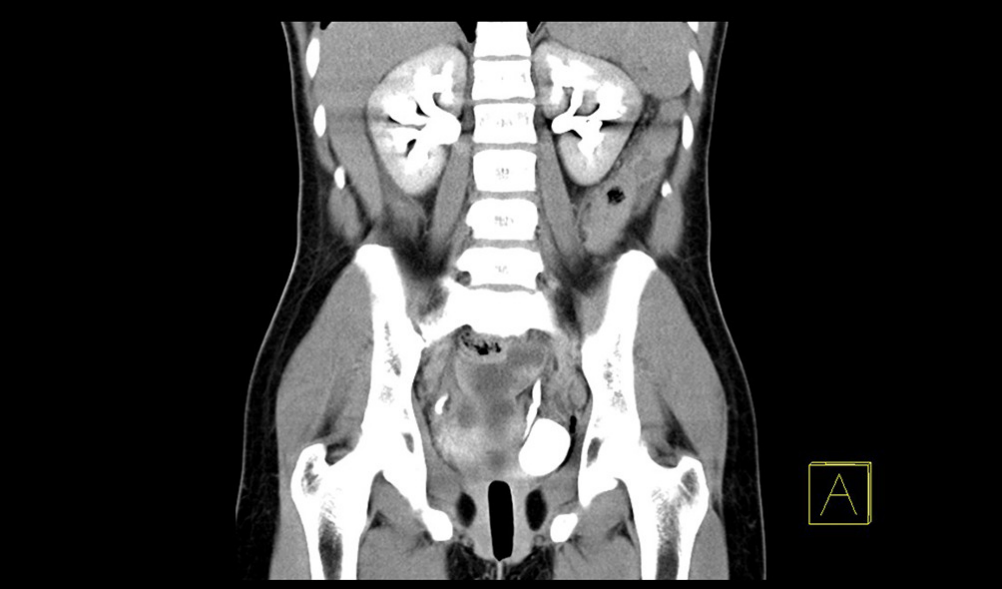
Delayed post-intravenous contrast CT scan (coronal reformat) showing the presence of a hypodense vaginal tampon for case 2.
